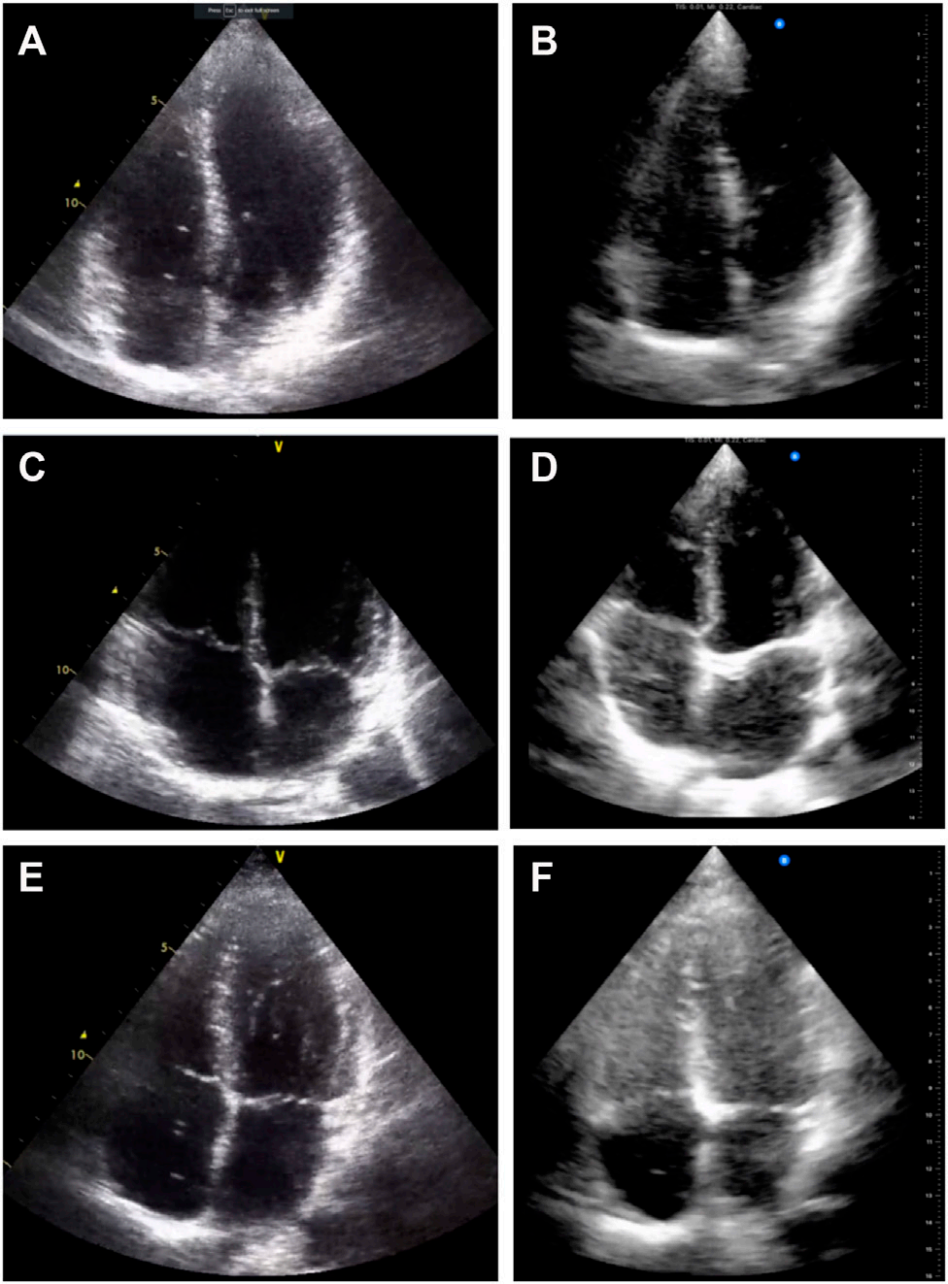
Example echocardiography images acquired with the Vivid q™ (A), (C), and (E) and with the Butterfly iQ™ (B), (D), and (F). Three different volunteers at the same measurement time point are presented for comparison (A–B), (C–D), and (E–F).
